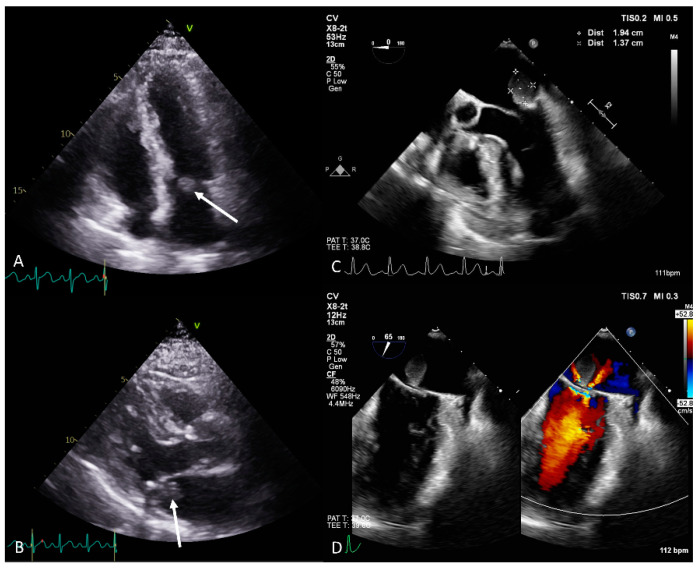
(A) Four-chamber transthoracic echocardiography view showing a mobile mass (white arrow) on the posterior leaflet of the mitral valve. (B) Parasternal long-axis transthoracic echocardiography view showing a mobile mass (white arrow) on the posterior leaflet of the mitral valve (Video S1). (C) Transesophageal echocardiography showing a 1.9 × 1.4 cm polypoid mass with a pedicle attached to the annulus near the P2 segment. The mass partially protruded into the left ventricle during diastole (Video S2). (D) Transesophageal echocardiography showing a polypoid mass with a pedicle attached to the annulus near the P2 segment. Color Doppler indicated mild mitral regurgitation. Grading of the mitral regurgitation may have been underestimated due to the mitral mass obstacle (Video S3). Preoperative coronary artery angiography showed no obvious feeding arteries (Videos S4–S6).
